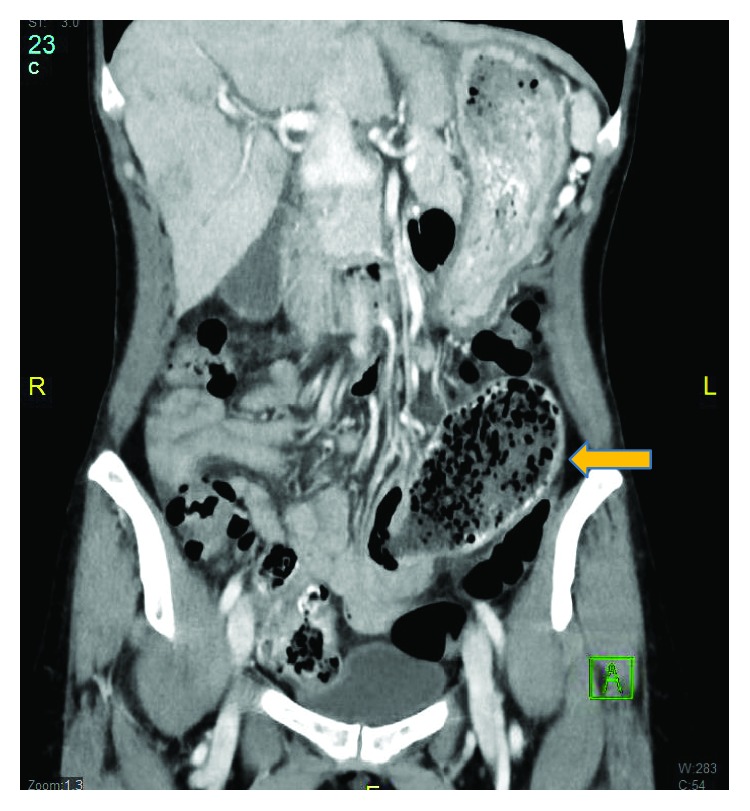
CT scan of the abdomen and pelvis showing a dilated jejunum and transition point.
